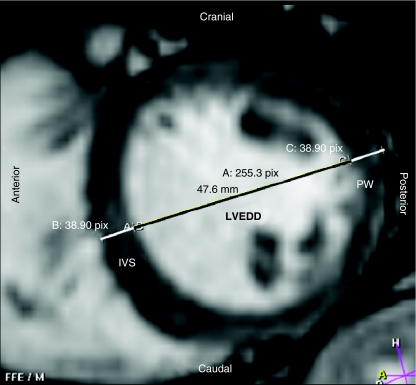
Measurements on short-axis magnetic resonance images were placed to correspond with measurements made on M-mode echocardiography and were used to calculate the left ventricular mass with the formula commonly used in echocardiography (LVM_MRI/ASE_). The end-diastolic inner left ventricular diameter (LVEDD) was measured from the middle of the inner aspect of the septal wall to the inner aspect of the posterior wall, and the interventricular septal thickness (IVS) and the posterior wall thickness (PW) were measured at the same level. To correspond with a parasternal short-axis M-mode view the image has to be turned 90 degrees clockwise.
